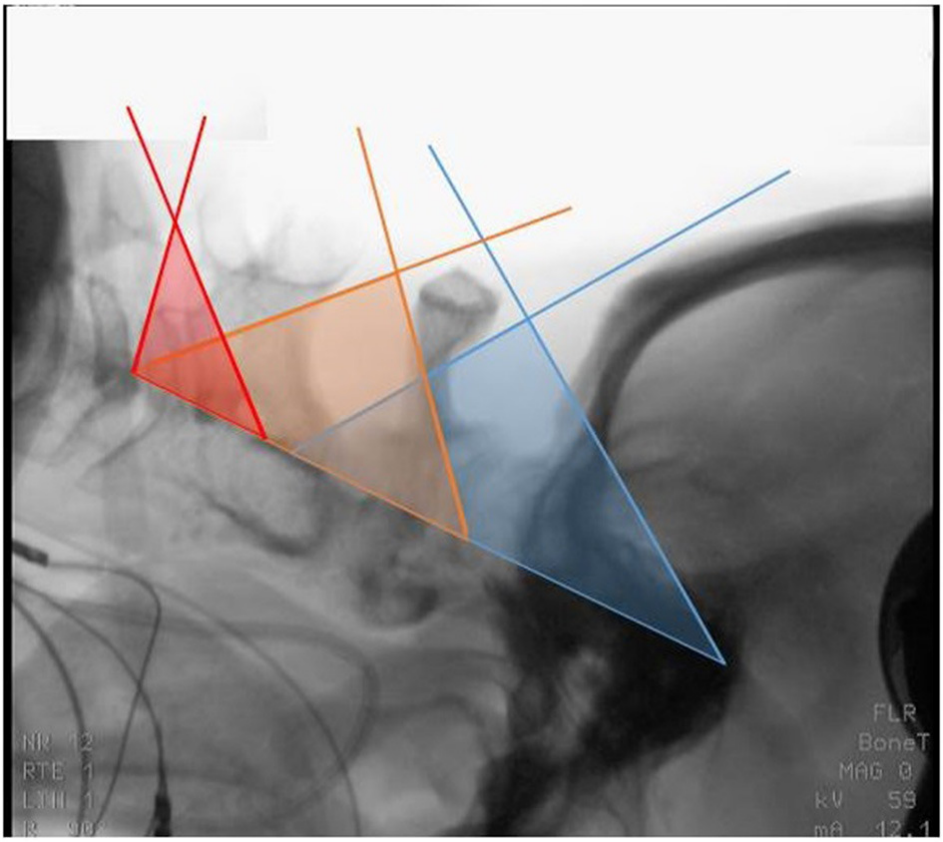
Lateral X-ray of case 4 after prone positioning with inclination. Colored sectors indicate the theoretical access supplied by the interspace between the occiput and the posterior arch of C1 (blue), C1 and the cranial edge of the lamina of C2 (yellow) and, at times, between the inferior edge of the lamina of C2 and the superior edge of the lamina of C3 (red).
